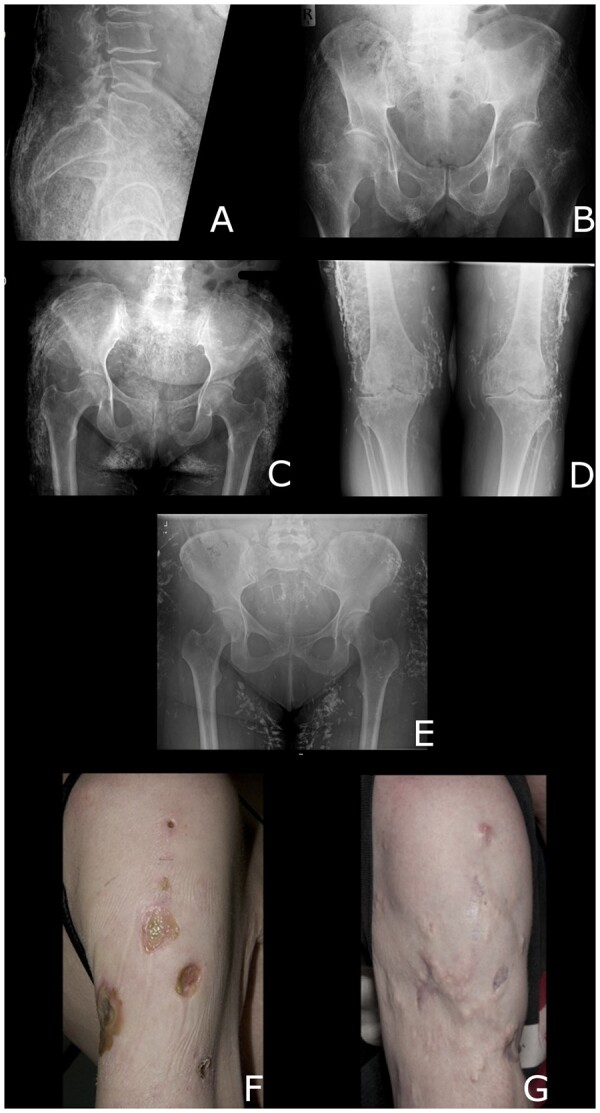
Plain radiographs (A–E) and photographic images (F, G) of calcinosis. (A, B) Calcinosis around the pelvis in patient 1. (C, D) Calcinosis around the pelvis and knees in patient 2. (E) Calcinosis around the pelvis in patient 3. (F, G) Areas of ulceration overlying calcinotic areas in the right upper arm of patient 4 (F) and (21 months later) the resulting disfigurement after healing (G)
